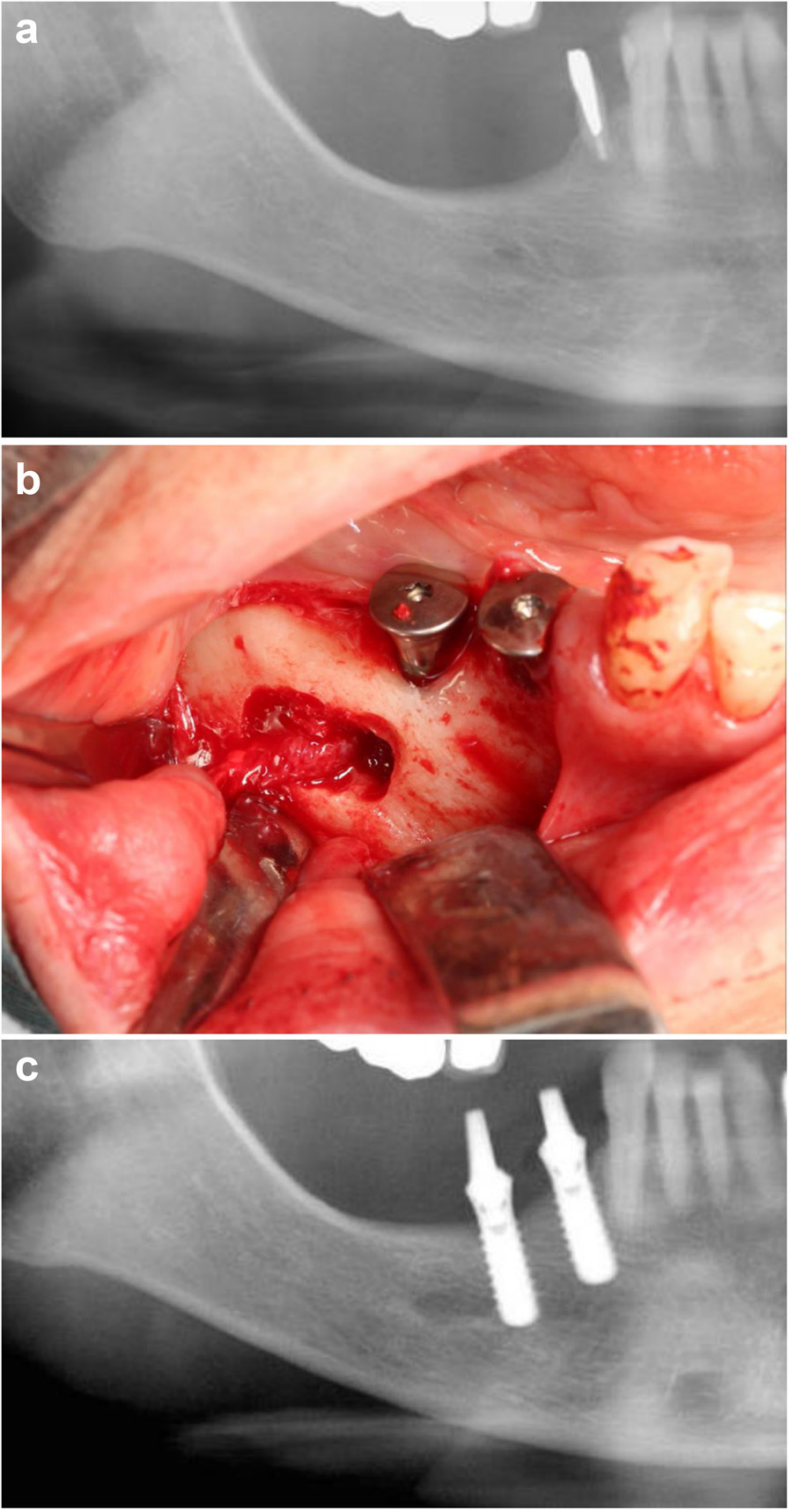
Alveolar nerve repositioning in a partially edentulous mandible. a Preoperative radiograph. b The inferior alveolar nerve was transposed from the mental foramen. c Postoperative radiograph after implant insertion
